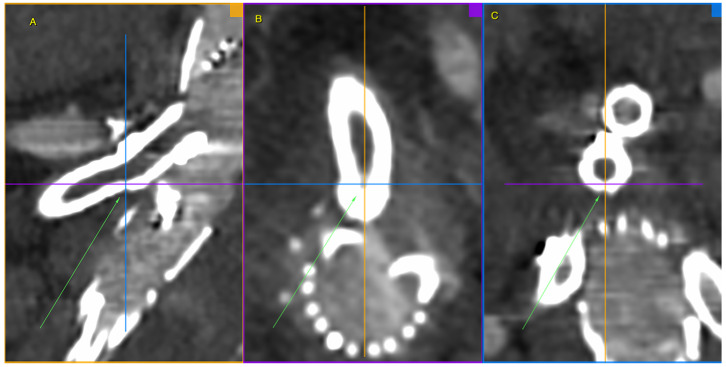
Repaired superior mesenteric artery branch of stent graft in three planes. The mended site is centrally located at the intersection of the lines defining the planes and additionally marked with green arrows. Panel (A)—sagittal projection, panel (B)—axial projection, panel (C)—coronal projection. Control Angio-CT 3D MPR, arterial phase.
